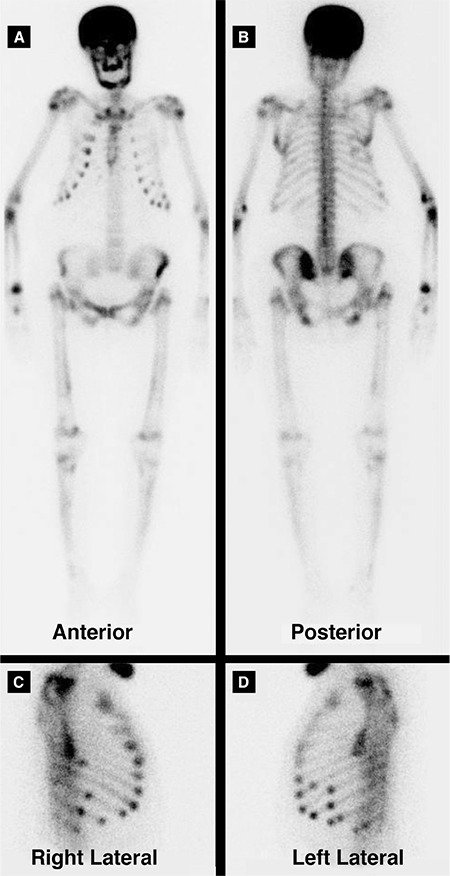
Tc-99m MDP bone scintigraphy. A 68-year-old women was admitted to the hospital on October 2013 with a mass on the cervical region. An excisional biopsy revealed parathyroid cancer. PET/CT showed multiple hypermetabolic lesions in the skeleton. The patient was treated by combination chemotherapy (including cisplatin and etoposide) plus zoledronic acid with good clinical response. Two years later, she presented with fatigue. Her laboratory results showed severe hypercalcemia and renal failure (serum calcium level 16.6 mg/dL, blood urea nitrogen/creatinine: 32/2.3 mg/dL). The parathormone level was 1859.6 pg/mL. Hydration was performed, calcitonin was prescribed but the hypercalcemia could not be controlled. Tc-99m MDP bone scintigraphy revealed intense radiotracer uptake involving almost the entire skeleton with high bone to soft tissue uptake (A and B). Bilateral kidneys were almost invisible (A and B). These findings are suggestive of ‘metabolic superscan’. There was focal radiotracer uptake in several ribs considered as Brown tumors according to CT findings (C and D). Brown tumors represent a reparative cellular process rather than a neoplastic process (1). The increased uptake of radiopharmaceutical by pathologic bone results in reduced phosphate excretion, thereby producing faint renal images on bone scintigraphy. Wiegemann et al. (2) reported that bone resorption, alkaline phosphatase level, and parathyroid hormone activity were not correlated with Tc-99m pyrophosphate uptake by the bone. Malignancies with known super scan in bone scintigraphy include prostate cancer, breast cancer, lung cancer, lymphoma, urinary tract transitional cell carcinoma, nasopharyngeal carcinoma and carcinoma of the colon (3,4,5). Hematologic conditions like leukemia, lymphoma, myelofibrosis, Waldenstrom’s macroglobulinemia have also been reported to be associated with super scan. This feature can also be detected in metabolic bone diseases like renal osteodystrophy, Paget’s disease and hyperparathyroidism (2)
